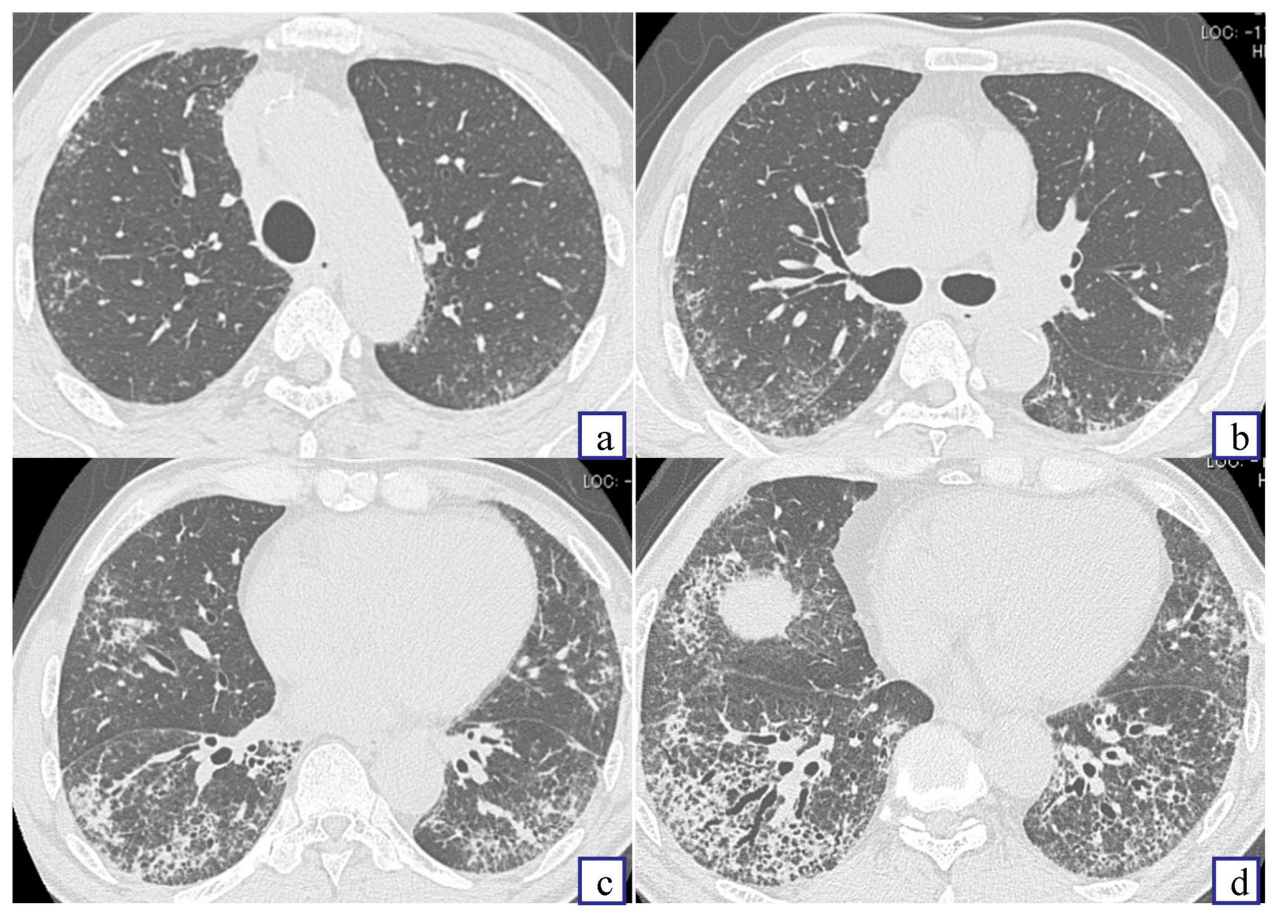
An example of high–resolution CT images. Analysis of each finding was as follows. Honeycombing: negative; Bronchiectasis: positive; Dilatation of pulmonary artery: negative; Extent of ground glass attenuation: grade 1; Extent of consolidation: grade 1; Extent of reticular abnormality: grade 2; Extent of micronodules: grade 2. This case was diagnosed as fibrotic nonspecific interstitial pneumonia pattern. (a) at the aortic arch. (b) at the carina tracheae. (c) at the right pulmonary vein. (d) at the top of the right diaphragm.
